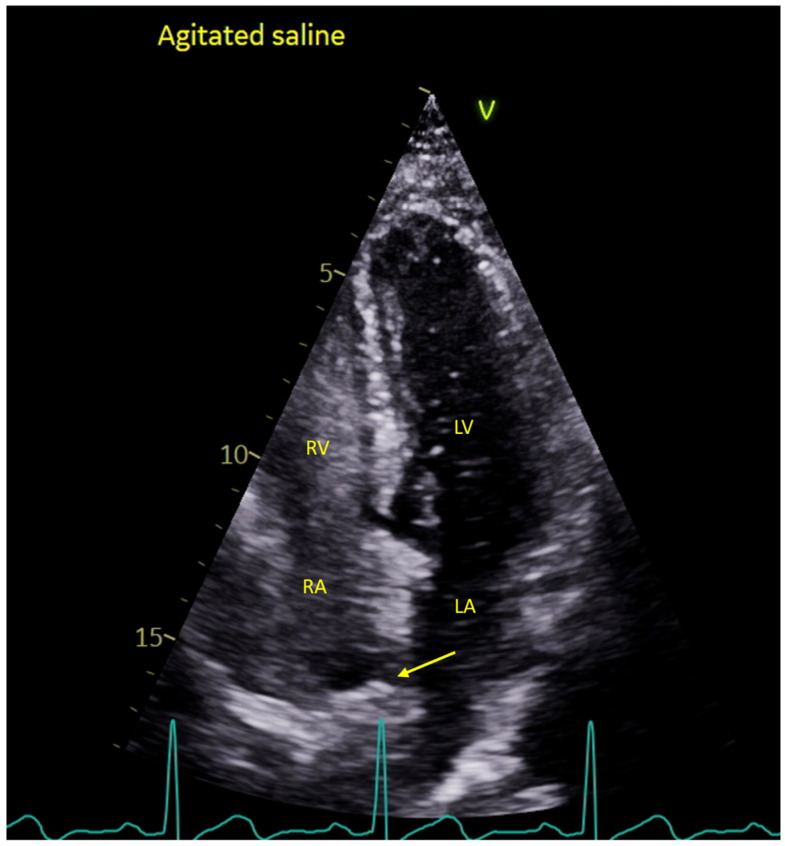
Transthoracic echocardiography with agitated saline demonstrating an intracardiac shunt and passage of bubbles from right-to-left (yellow arrow). Transthoracic echocardiography (TTE) was performed to evaluate the presence of an intracardiac shunt using agitated saline contrast (“bubble study”). Echogenic microbubbles do not traverse the pulmonary circulation; therefore, their appearance in the left-sided cardiac chambers indicates a right-to-left shunt [6]. Intracardiac shunts represent a potential mechanism for paradoxical embolism, whereby thrombotic material originating in the venous circulation gains access to the systemic arterial circulation, including the posterior cerebral circulation [7]. In this case, her TTE demonstrated normal left and right ventricular size and systolic function. There was early right-to-left passage of agitated saline on the apical four-chamber view within three cardiac cycles (Figure 2; Video S1), consistent with the presence of an intracardiac shunt. Formal screening for venous thrombosis, including lower limb Doppler ultrasound or D-dimer testing, was not performed during the admission. Nevertheless, the postoperative state following recent surgery was considered a potential prothrombotic condition. The differential diagnosis for right-to-left shunting identified by contrast echocardiography includes patent foramen ovale (PFO) as the most common cause, followed by atrial septal defects and, less frequently, pulmonary arteriovenous malformations [8]. Although there is no formal guideline consensus regarding the sensitivity and specificity of TTE with agitated saline, it is widely recommended as the preferred initial screening modality over two-dimensional transthoracic echocardiography with color Doppler alone for the detection of right-to-left shunts [6]. Assessment of thrombosis risk factors is important in patients with an intracardiac shunt, as such defects may permit embolic material originating in the venous circulation to enter the systemic arterial circulation while bypassing pulmonary filtration. In this patient, several relevant prothrombotic risk factors were present, including obesity (body weight 107 kg), heavy tobacco use (approximately 40 cigarettes per day), and a history of hypertension. However, she had no prior history of venous thromboembolism or cancer. LA = left atrium; LV = left ventricle; RA = right atrium; RV = right ventricle.
